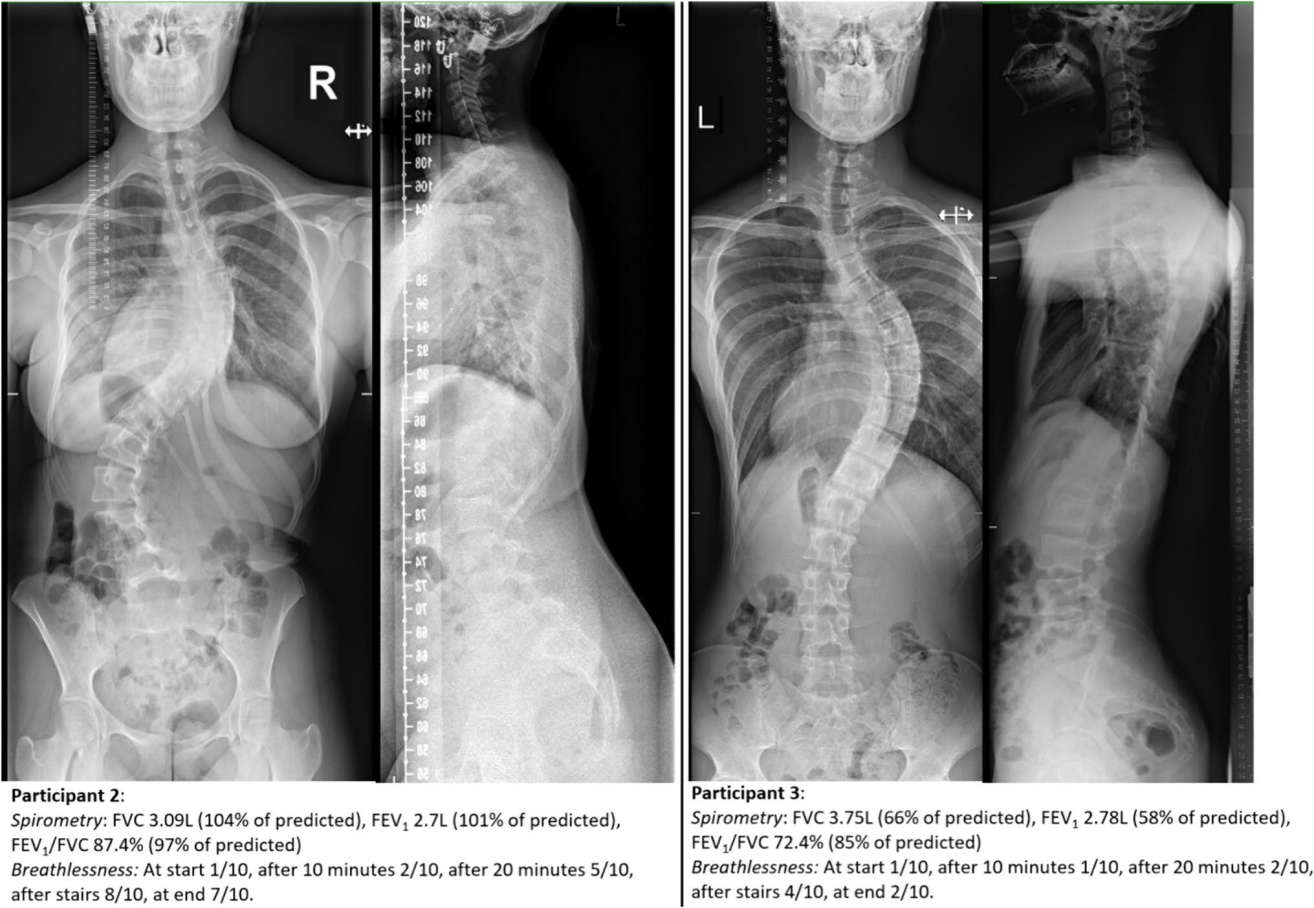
Posteroanterior (PA) and lateral full spine radiographs of participant two and three with similar curve magnitude and hypokyphosis, demonstrating markedly different spirometry and breathlessness measurements. Participant two has normal spirometry yet experiences significant breathlessness during exercise, whereas participant two demonstrates markedly reduced spirometry results but very little breathlessness during exercise
